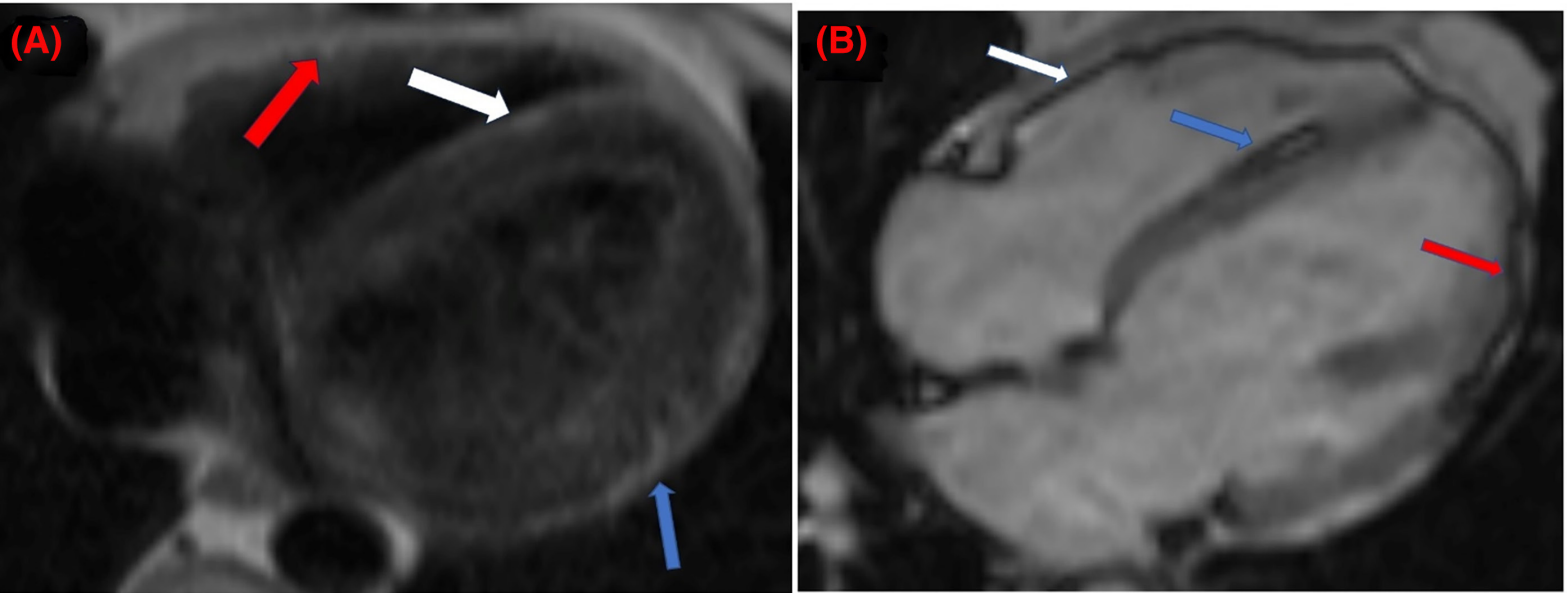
(A) Cardiac MRI half‐fourier single‐shot turbo spin‐echo (HASTE) axial sequence showing increased signal intensity along the right ventricular free wall (red arrow), interventricular septum (white arrow), and epicardial lateral left ventricular wall (blue arrow). (B) Cardiac MRI 4 chamber balanced steady‐state free precession (bSSFP) scout showing chemical artifact on right ventricular free wall (white arrow), interventricular septum (blue arrow), and epicardial lateral left ventricular wall (red arrow).
